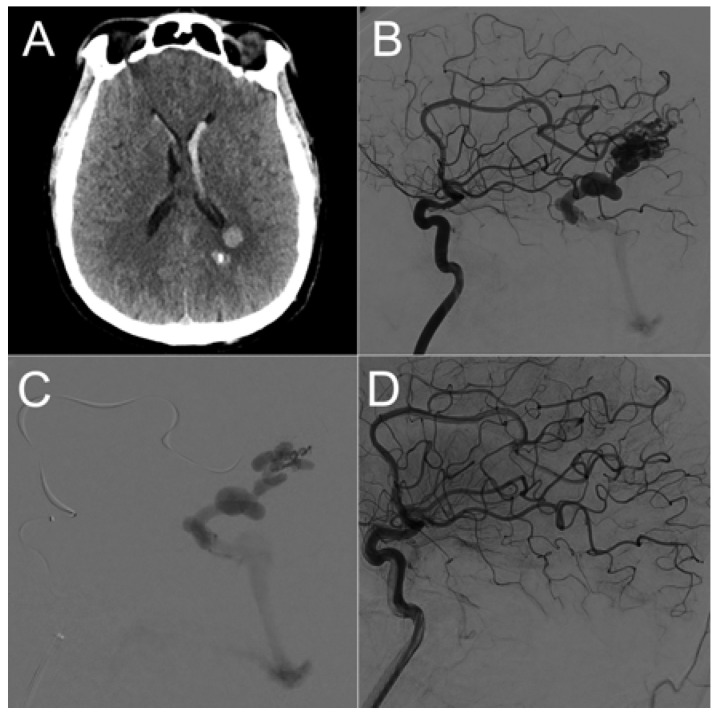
Male patient in his 40s presenting with acute onset of headache. Emergency brain CT scan (A) revealed left parietal intraparenchymal hematoma and intraventricular hemorrhage. Digital subtraction angiography of the left internal carotid artery (ICA) lateral projection (B) showed an underlying left parietal bAVM. The two main arterial feeders originated from the pericallosal artery. Endovascular embolization was performed via distal microcatheterization of the feeders and ultra-selective angiography (C) followed by an injection of the embolic product (Onyx 18, Ev3 Neurovascular, Irvine, CA, USA). The final angiographic of the left ICA (D) shows complete bAVM exclusion.
